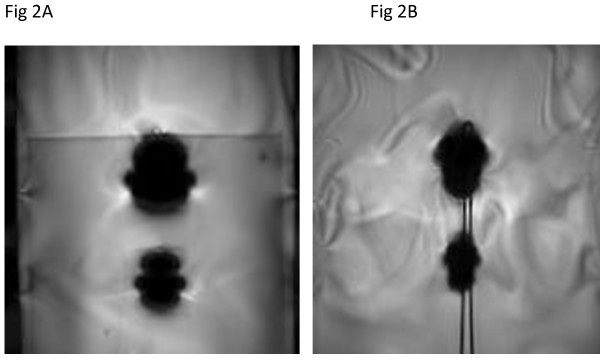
MRI artifacts. MRI artifacts associated with the skin closure staple (bottom) and hemostatic clip (top); (a) long axis and (b) short axis imaging planes (GRE pulse sequence; TR/TE 100-msec/15-msec; flip angle, 30 degrees; field of view, 26-cm).
